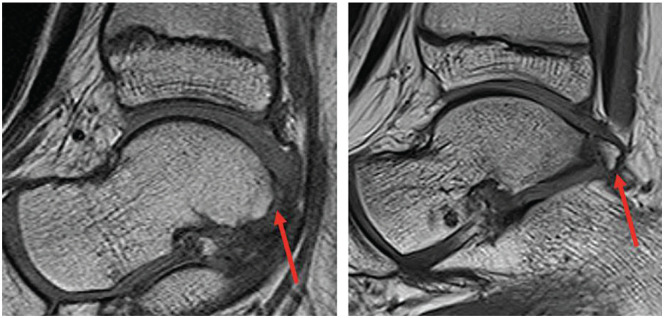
An OT is visualized in the sagittal T1-weighted MRI on the right, compared with a similar slice from an ankle MRI of a patient without an os trigonum (left). MRI, magnetic resonance image; OT, os trigonum.
